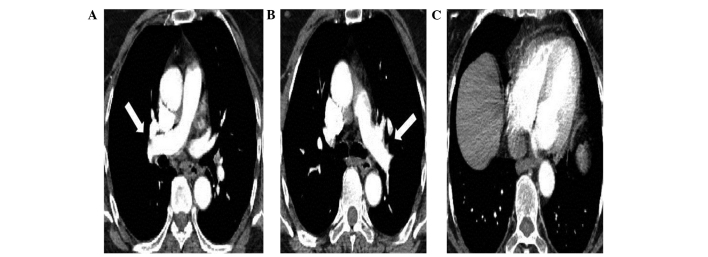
Computed tomography scan following thrombolytic therapy. (A) Reperfusion of the branches of the (A) right and (B) left pulmonary arteries. (C) Normalization of the diameter of the left and right ventricular cavities.
